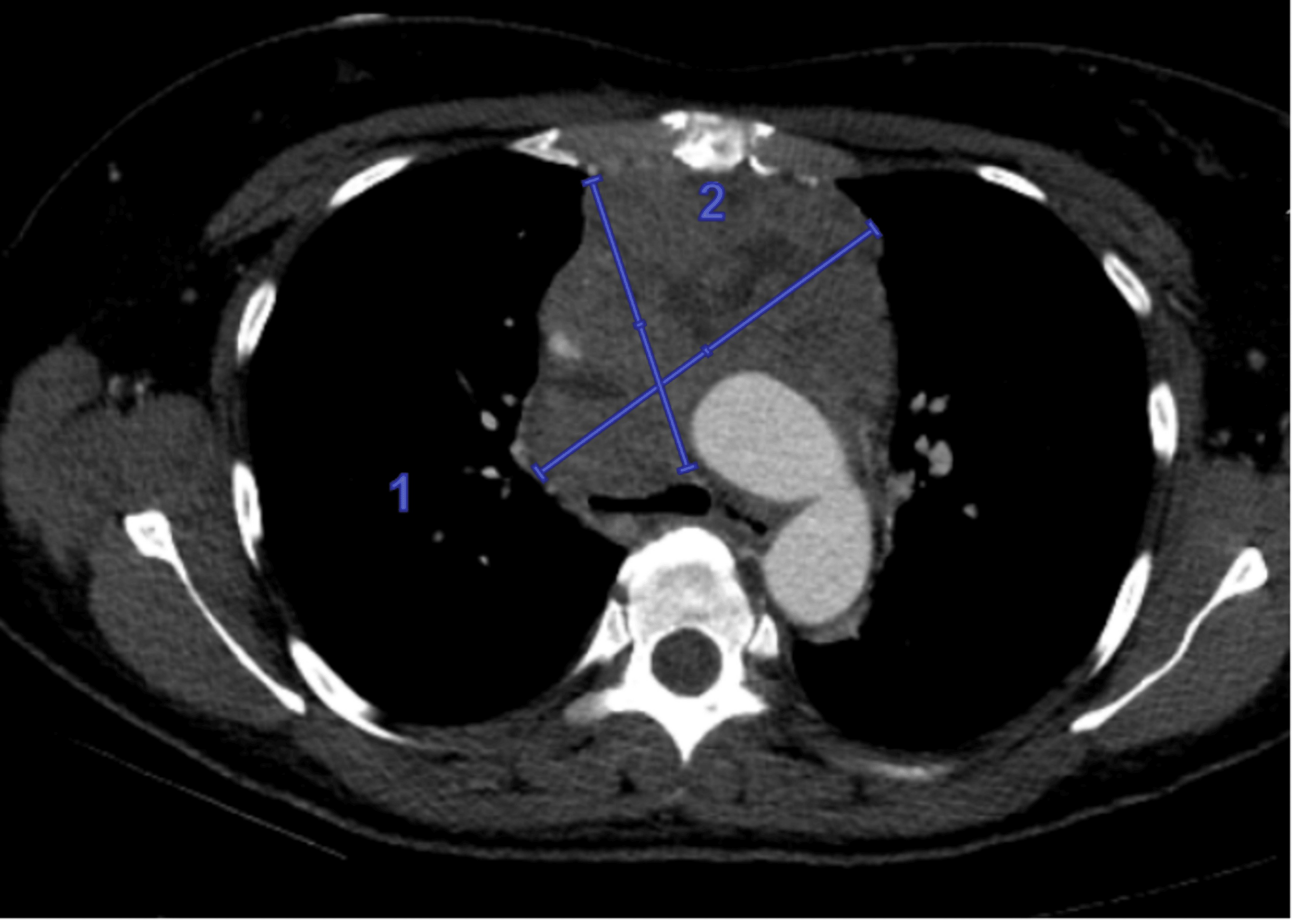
Large superior mediastinal mass seen on contrast-enhanced axial computed tomography (blue lines numbered 1 and 2, approximately 10 × 7.3 × 12.3 cm) with the extensive involvement of vascular structures (the superior vena cava, brachiocephalic vein, and ascending aorta) and adjacent airways (the trachea and right main bronchus), consistent with malignancy.
